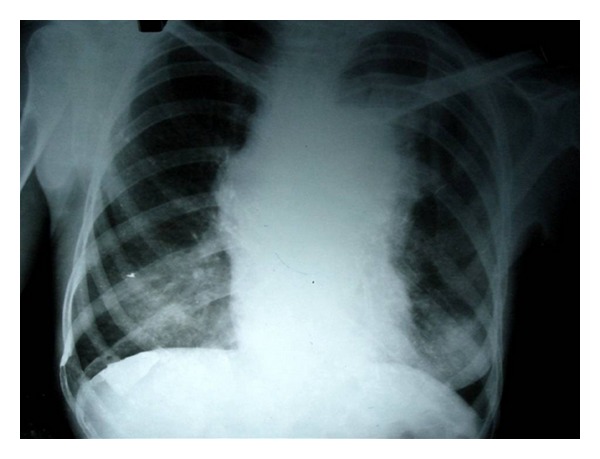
X-ray chest (PA view) showing a mediastinal mass.
